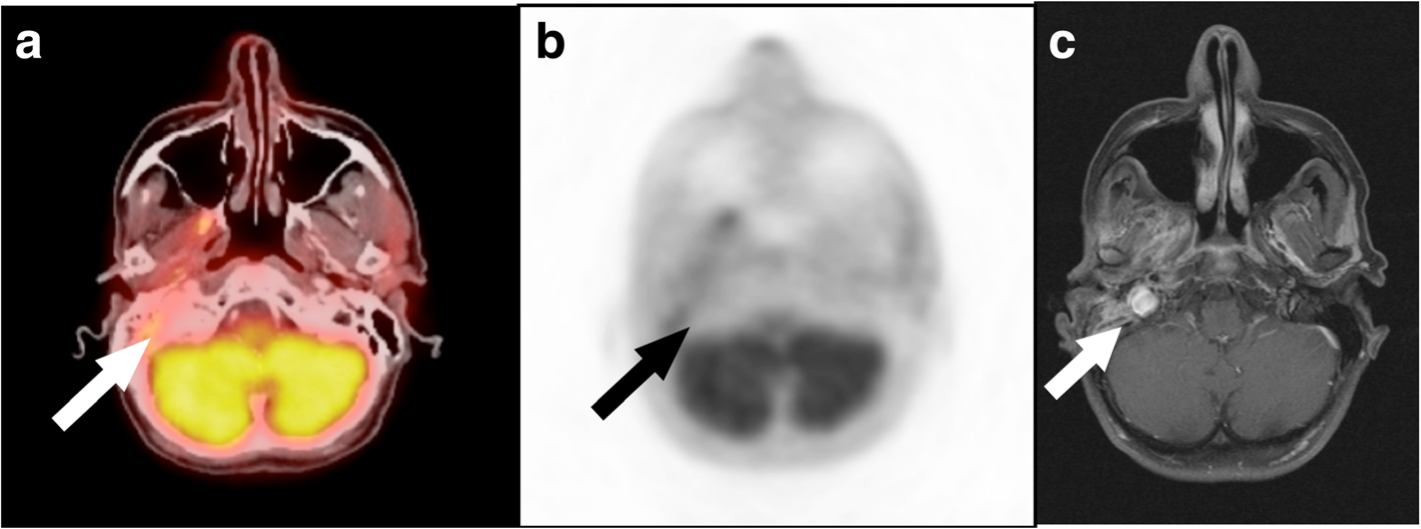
ACC recurrence: PET/CT imaging was false-negative and MRI true-positive. Patient after multiple resections of the primary and recurrent tumours of an ACC in the right parotid gland area. A false-negative finding is seen on the on the fused PET/CT (a) and PET image (b), but there was a true-positive finding on the contrast-enhanced T1-weighted MR image (c) with a hyperintense tumour recurrence (arrow)
